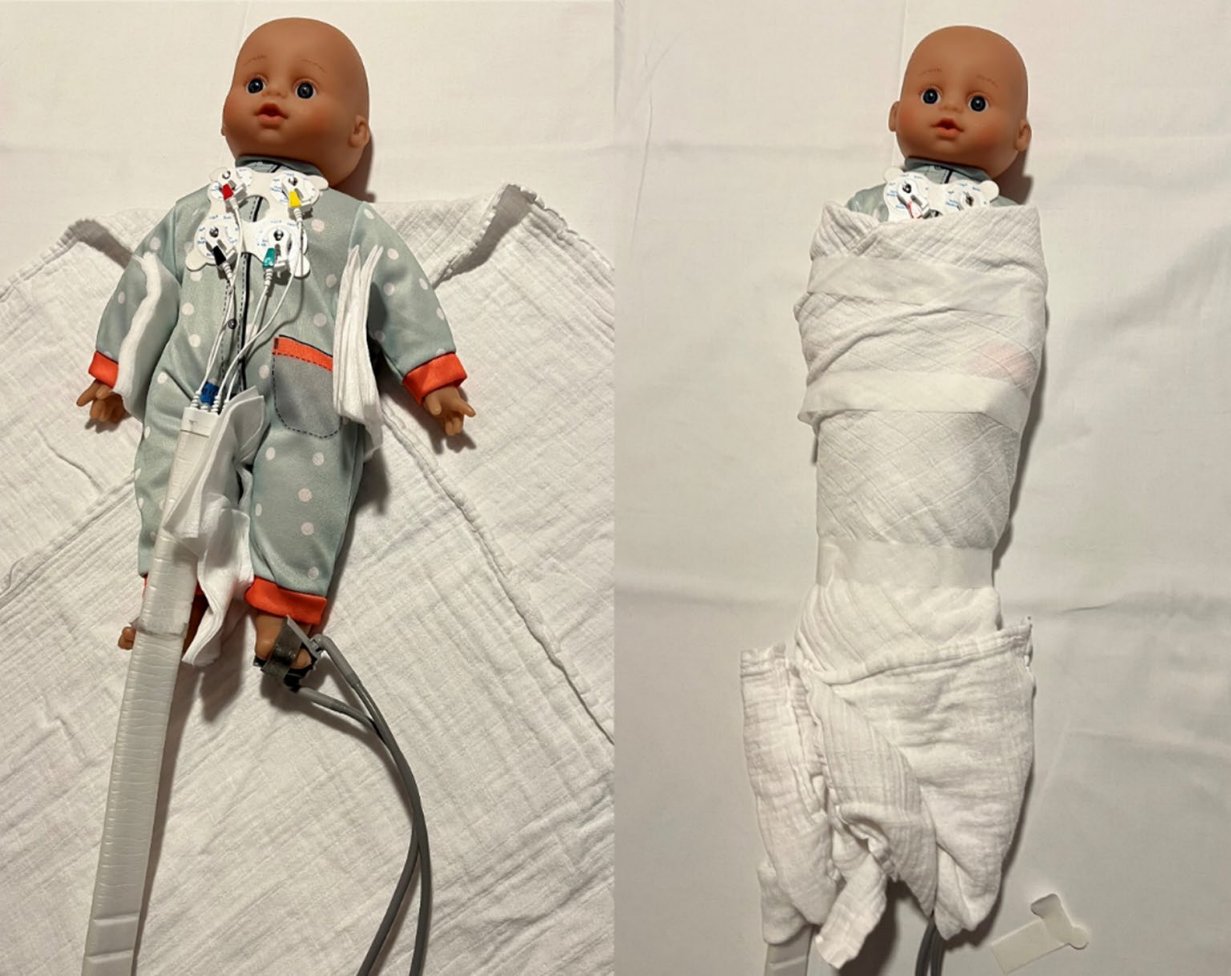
An impression of the swaddle technique, which is applied prior to scanning, to obtain an MRI scan of infants without general anaesthesia. The infant is first fed by one of the parents or caregivers. After feeding, an MRI technician applies equipment to monitor heart rate and blood pressure throughout the scan. Pieces of cloth are placed in between the legs and arms, to prevent skin from touching. The MRI technician then tightly swaddles the infant using (thin) blankets. This will encourage the child to fall asleep and remain still during the scan. Earplugs, a headphone or cushions can be used to protect from the loud scanning noises
